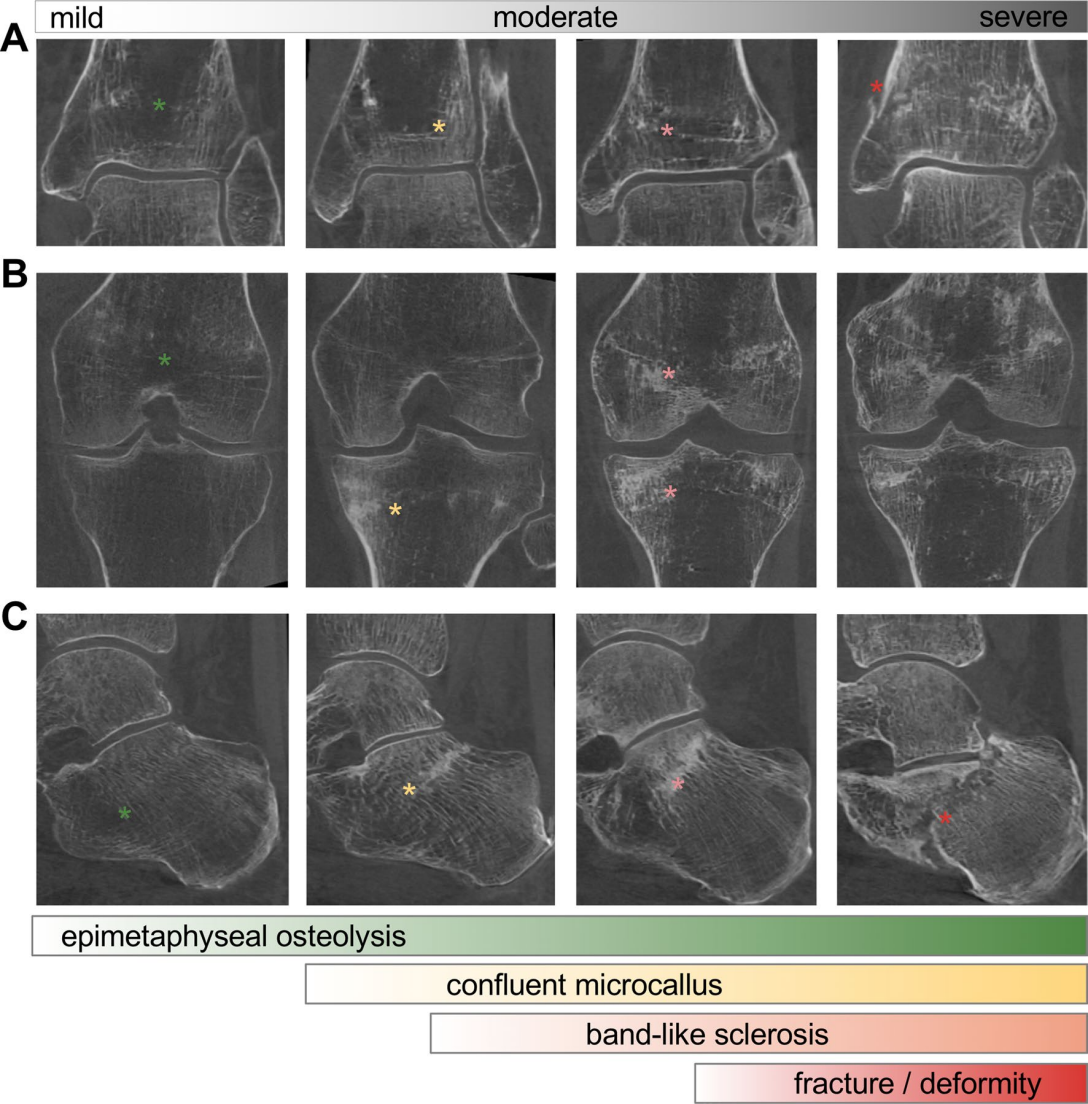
Cone beam CT (CBCT) imaging. Differentiation and classification of different disease severities based on CBCT imaging a in the distal tibia, b around the knee (distal femur and proximal tibia) and c in the calcaneus
